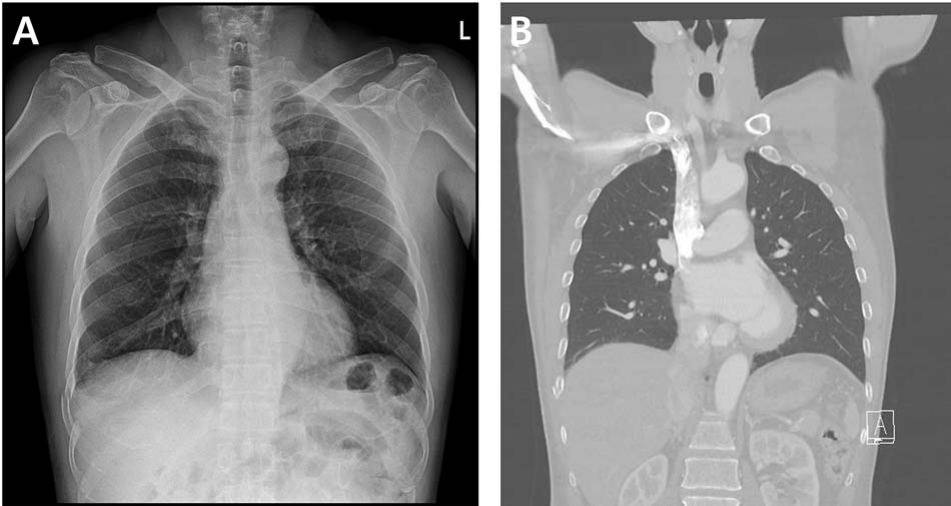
Radiographic findings. (A) Initial chest X-ray showed no evidence of hemothorax. (B) Initial chest CT also showed no evidence of hemothorax.
